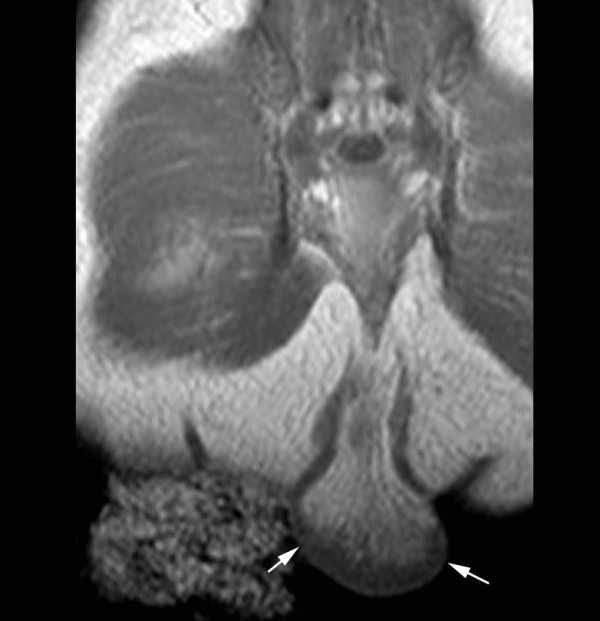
Magnetic resonance imaging of pseudotail. This T1 coronal image through the sacrum demonstrates the fat containing pseudotail (arrows).
